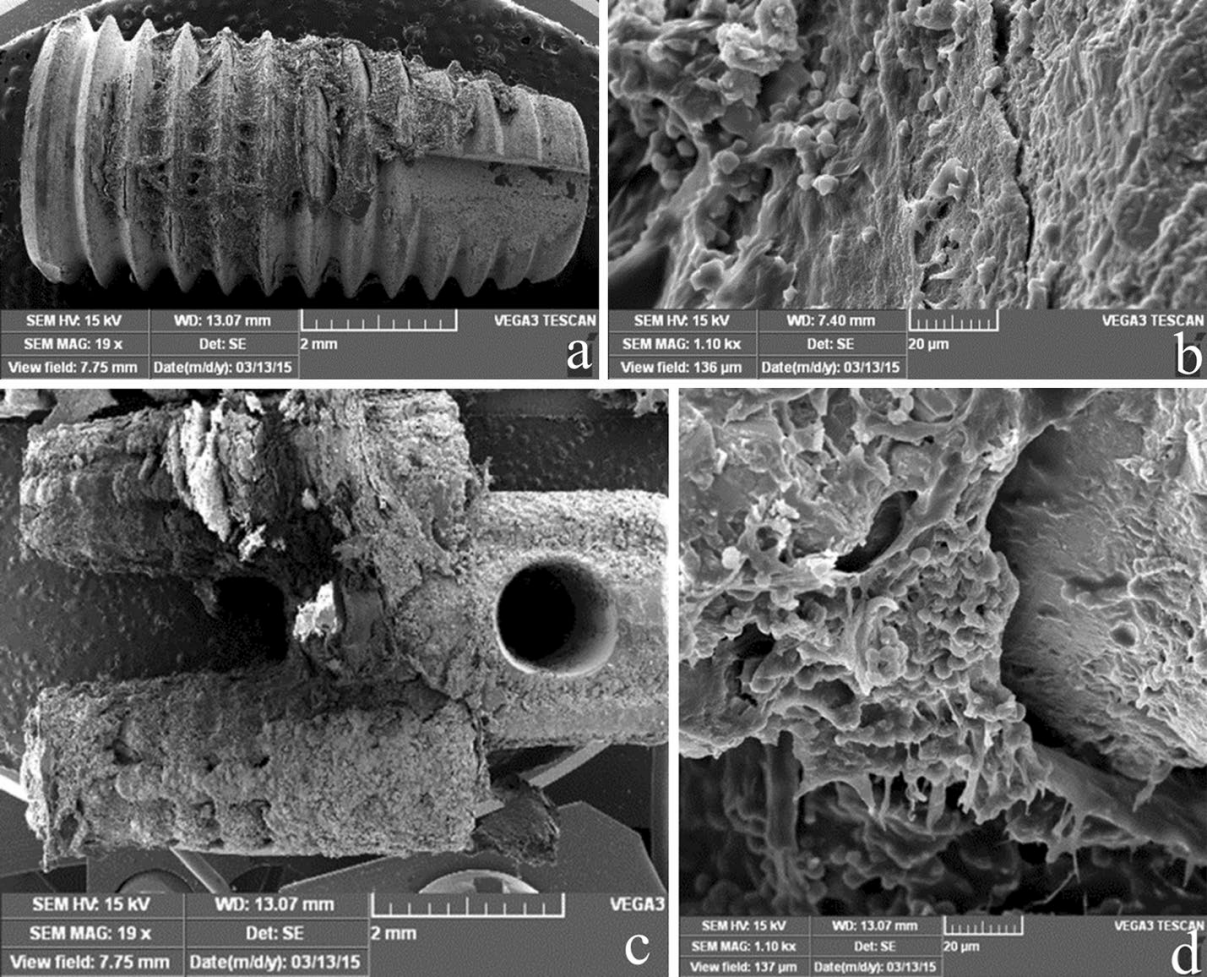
SEM images of the torque test-removed implants 8 weeks after implantation. a A global image of an RBM implant, b high-resolution image (×1.1 K) of an RBM implant, c global image of an MRI, d high-resolution image (×1.1 K) of an MRI implant
